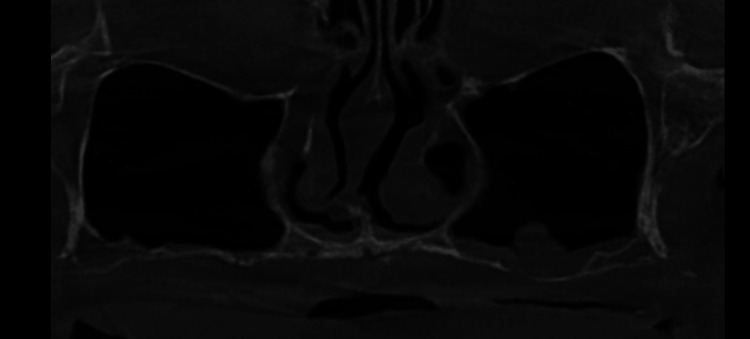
Panoramic reconstruction of CBCT exam. Notice extreme bony atrophy with less than 1 mm bone height under the maxillary sinus and the pterygomaxillary area with basal bone still available
